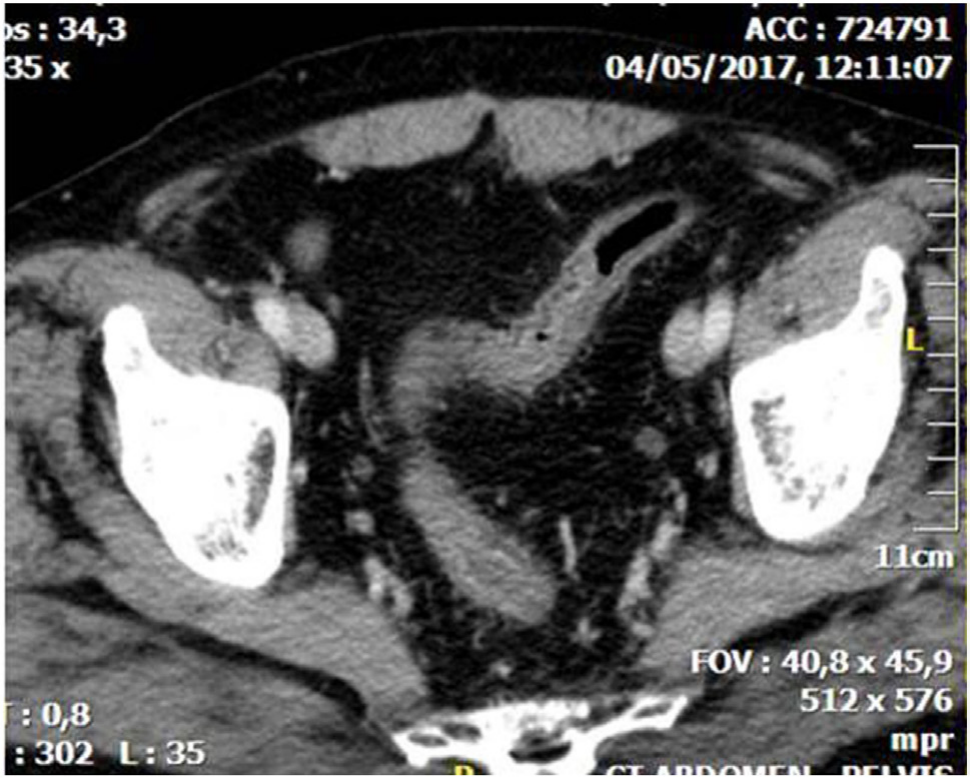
Abdominal CT showing diffuse thickening with layering of the wall of the sigmoid due to whole thickness ischemia.
